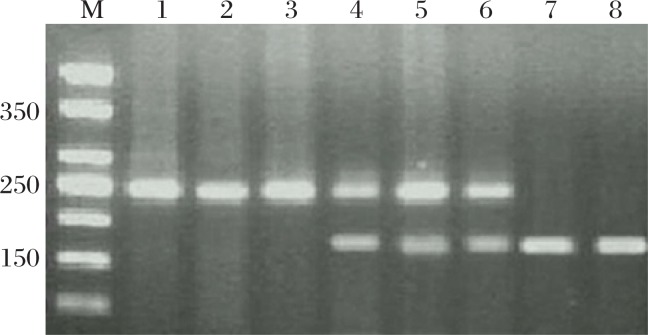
MTHFR* C 677T gene fragment length polymorphism distribution by *Hin*f I restriction enzyme (2.5% agarose gel electrophoresis). M: 100 bp ladder Marker; Lanes 1-3: CC genotype; Lanes 4-6: CT genotype; Lanes 7 and 8: TT genotype.
